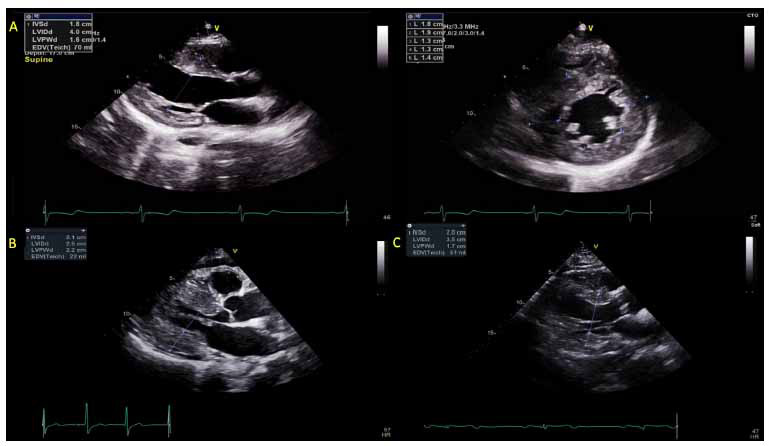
TTE images representing patterns and severity of LVH in the phenotypic expressions of HCM. (A) (row) = parasternal long (top left) and short (right) axis views of a patient with concentric/neutral HCM. (B and C) = parasternal long axis views of more severe hypertrophy in two patients with HOCM.
